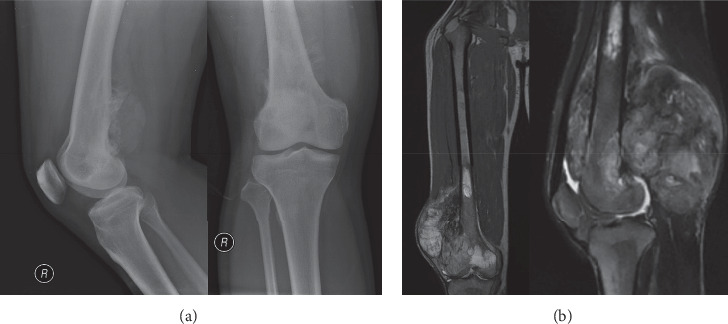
Radiographical appearance of parosteal osteosarcoma showing radiodense sessile lesion on the bone cortical surface, localized in the popliteal fossa (a). Magnetic resonance of T2-weighted coronal and sagittal images (b) depicting the heterogenic solid mass of distal femoral epimetaphysis with posterolateral soft tissue expansion. High signal intensity on the proximal marrow suggested intramedullary metastasis.
